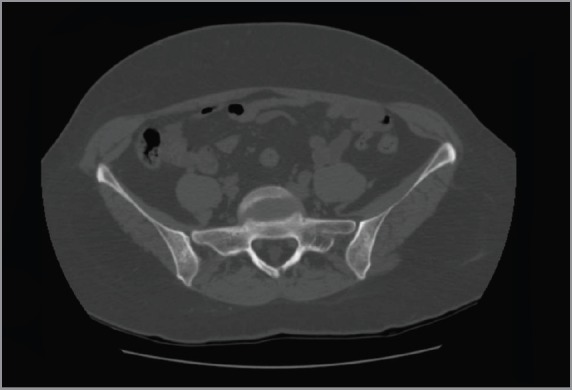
CT of the pelvis. Preliminary CT scan before CT-guided biopsy showed no evidence of abnormalities or where to target the biopsy.
